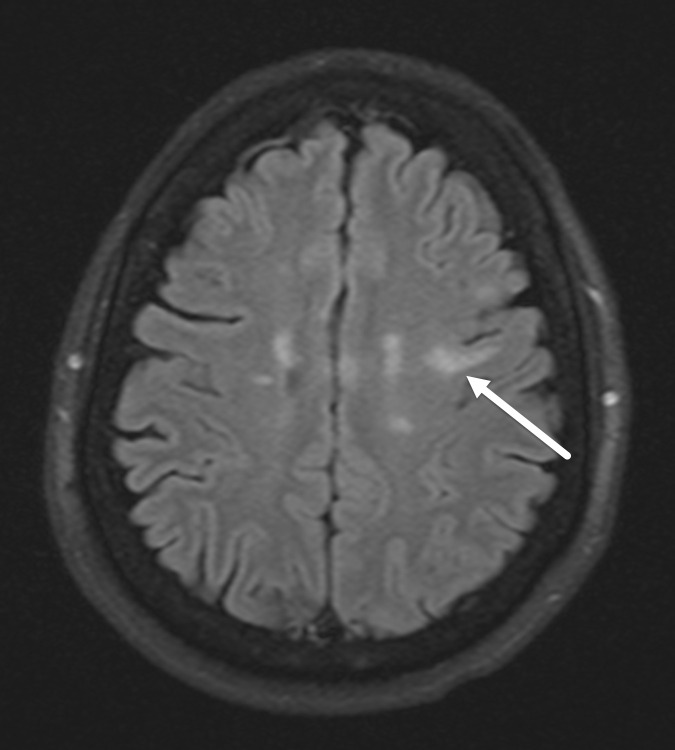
Partial resolution of the left frontal lobe lesion on follow-up. Axial FLAIR image demonstrates partial resolution of the left frontal lobe lesion at 4 month follow-up, formally excluding neoplasm as a differential. FLAIR, fluid attenuated inversion recovery.
